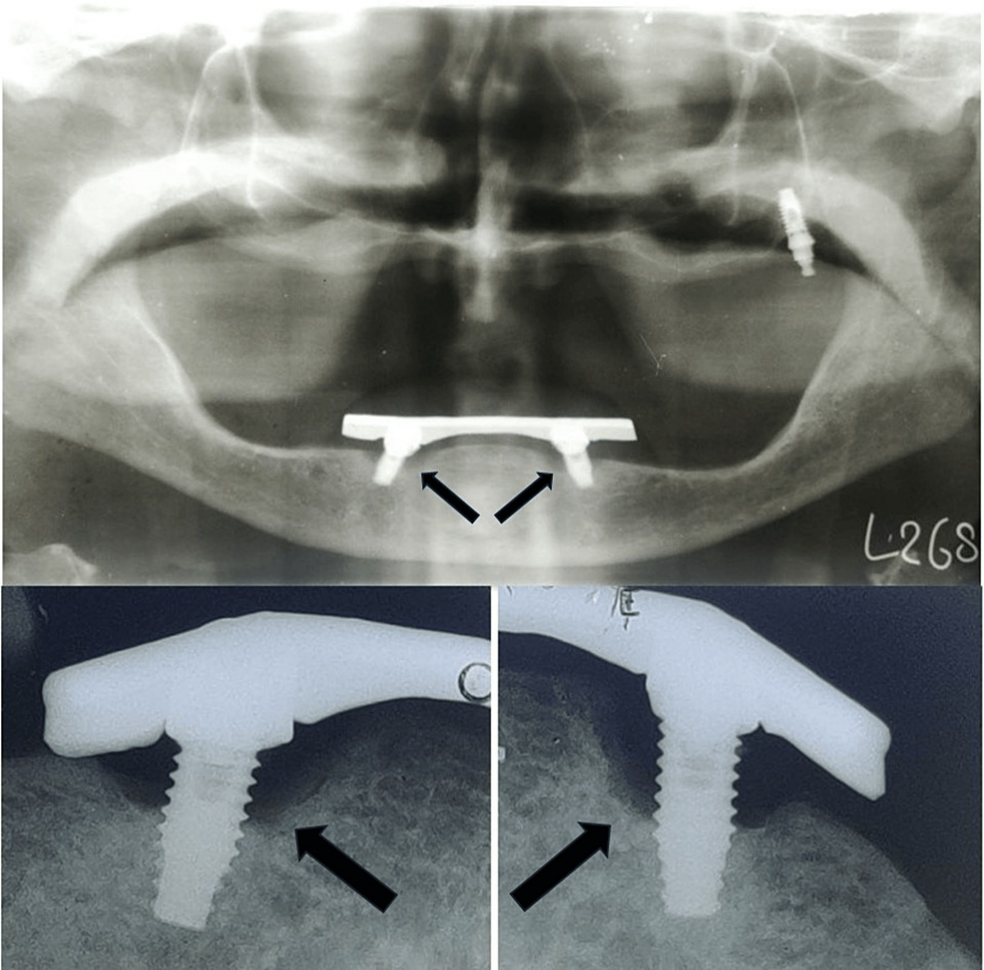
One-year follow-up radiographs showing no bone loss Arrows showing stable bone levels around mandibular implants
